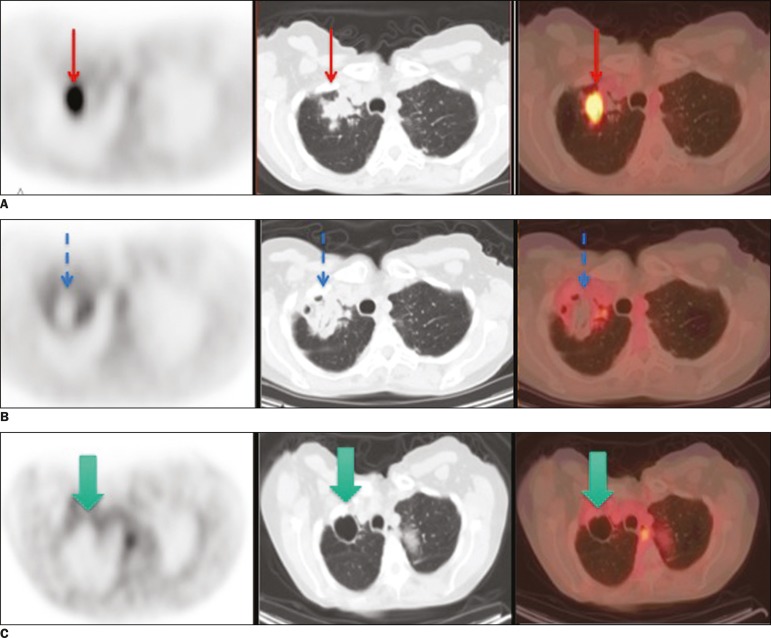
A: Baseline ^18^F-FDG PET/CT images of a lung
